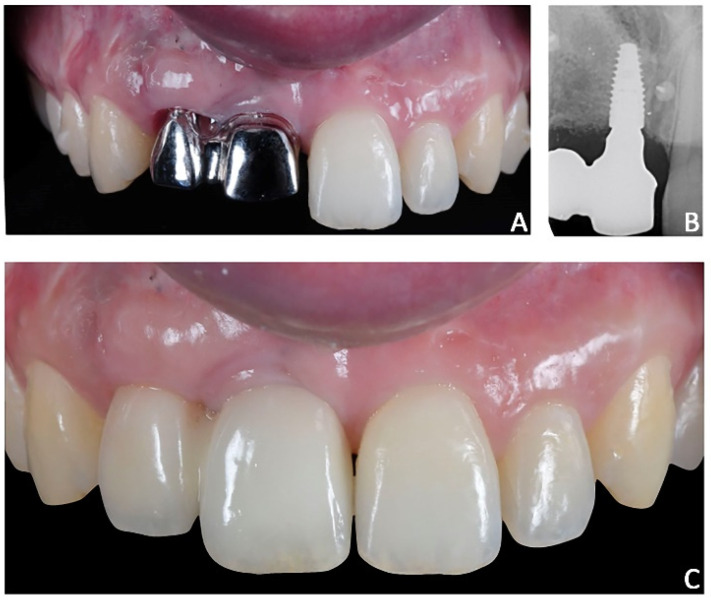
Case 2. Final prosthesis using milled cobalt-chrome structure and feldspathic porcelain. (A) Milled cobalt-chrome structure; (B) radiographic evaluation; (C) prosthesis veneered with feldspathic porcelain.
